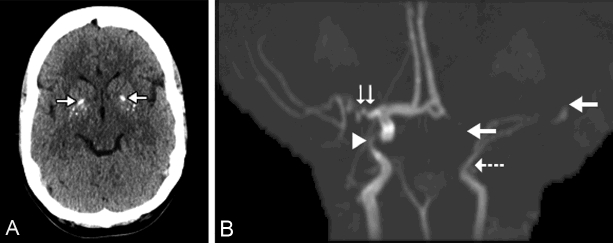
Radio logic findings in two patients homozygous for the Arg164X mutation in *SAMHD1*. (A) Patient 3 at age 25 years: Calcifications (arrows) in the basal ganglia (CT). (B) Patient 4 at age 14 years: MR angiography showing stenosis of the left distal internal carotid artery (arrow with dashed line) and discontinuation of the left medial cerebral artery (upper full arrow). The A1 segment of the left anterior cerebral artery is not detectable (lower full arrow). On the right, severe stenoses of the internal carotid artery (white arrowhead) and of the proximal part of the medial cerebral artery (double vertical arrow).
